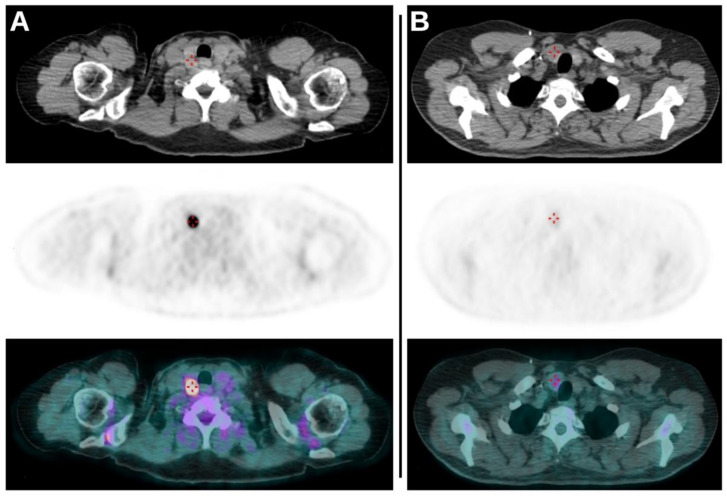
(A): Axial CT, axial PET and axial fused PET/CT images demonstrating the presence of TI revealed as intense focal uptake of ^18^F-FDG on the right lobe of thyroid. The lesion had a SUVmax of 44.47, an MTV of 0.7 and a TLG of 18.1 and subsequent cytological exam revealed no malignancy (TIR2). (B): Axial CT, axial PET and axial fused PET/CT images of another scan demonstrating again the presence of TI as a faint uptake on the right lobe of thyroid. The values of SUVmax, MTV and TLG of the lesion were 2.64, 6.9 and 10.3, respectively. Cytological evaluation (TIR5) and subsequent total thyroidectomy revealed the presence of papillary carcinoma.
